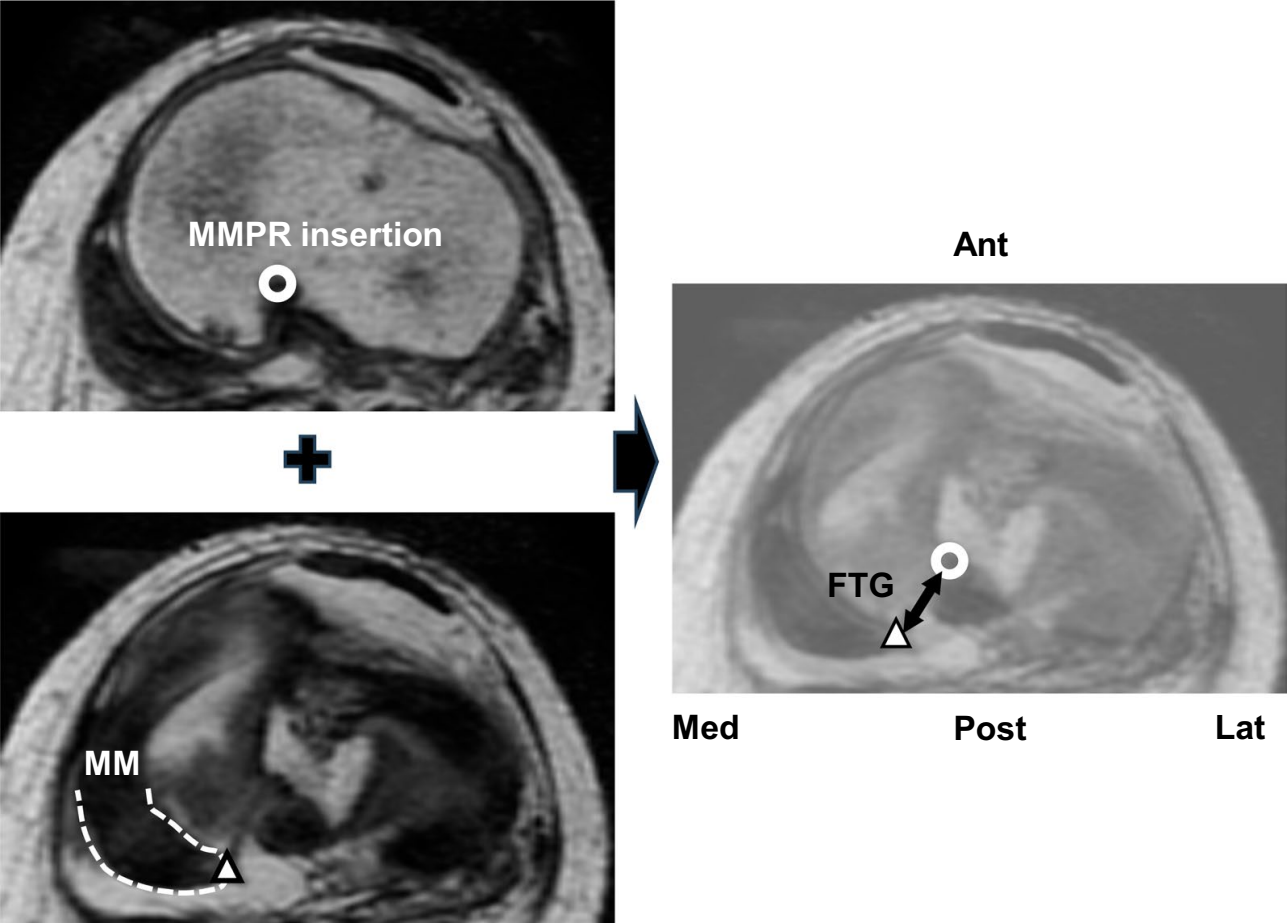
Magnetic resonance imaging measurement of the flexion tear gap (FTG). Image overlay of two axial magnetic resonance images of the knee flexed at 90° shows the FTG. The FTG was measured as the distance between the medial meniscus (MM) posterior root (MMPR) insertion (center of the white circle) and the tip of the tear stump (triangle)
